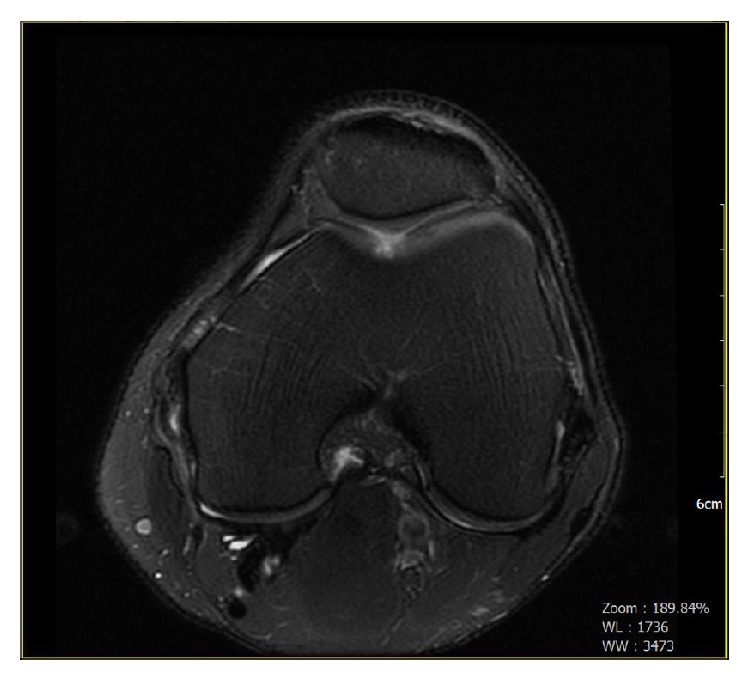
Preoperative radiograph. Multiplanar, multisequential images were obtained through the left knee on a 1.5 Tesla MRI scanner. Mild patellofemoral joint osteoarthritis. A focal region of grades III-IV chondromalacia overlying the central femoral trochlear groove.
